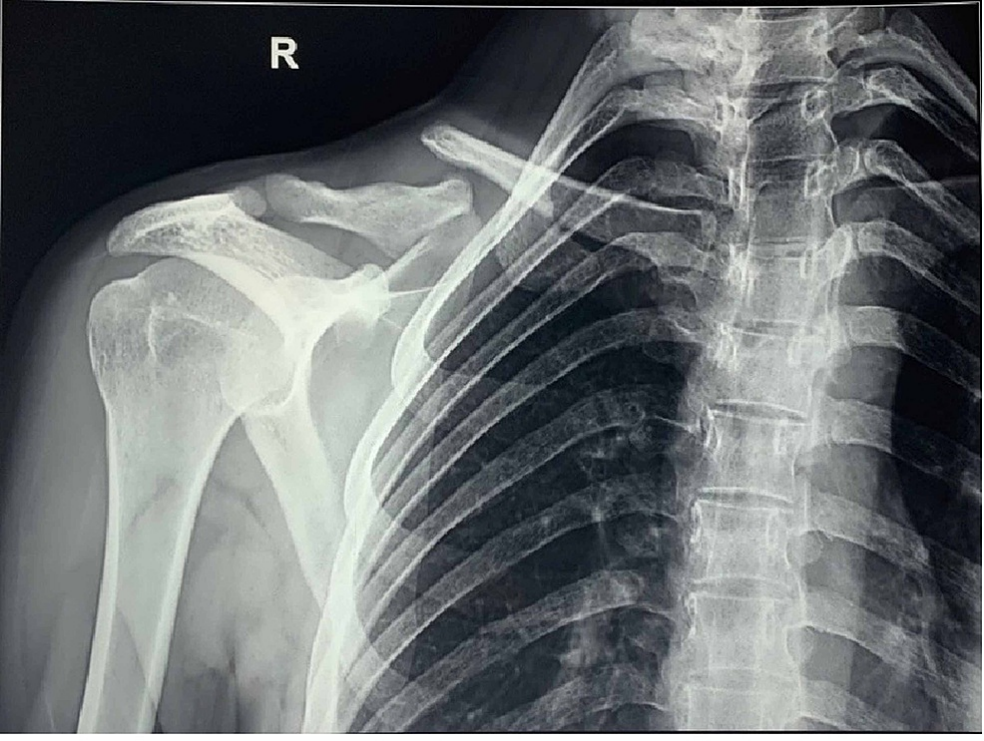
Radiograph of the conservatively treated right clavicle fracture showing non-union
